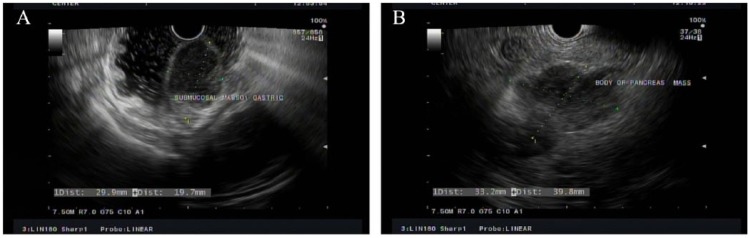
(A) Endoscopic ultrasound (EUS) showing the gastric mass arising from the submucosal gastric layer, measured 29.9 × 19.7 mm in diameter. (B) EUS showing an irregular, well-defined, and heterogeneous solid mass with cystic components in the body of the pancreas measured 33.2 × 39.8 mm in diameter.
